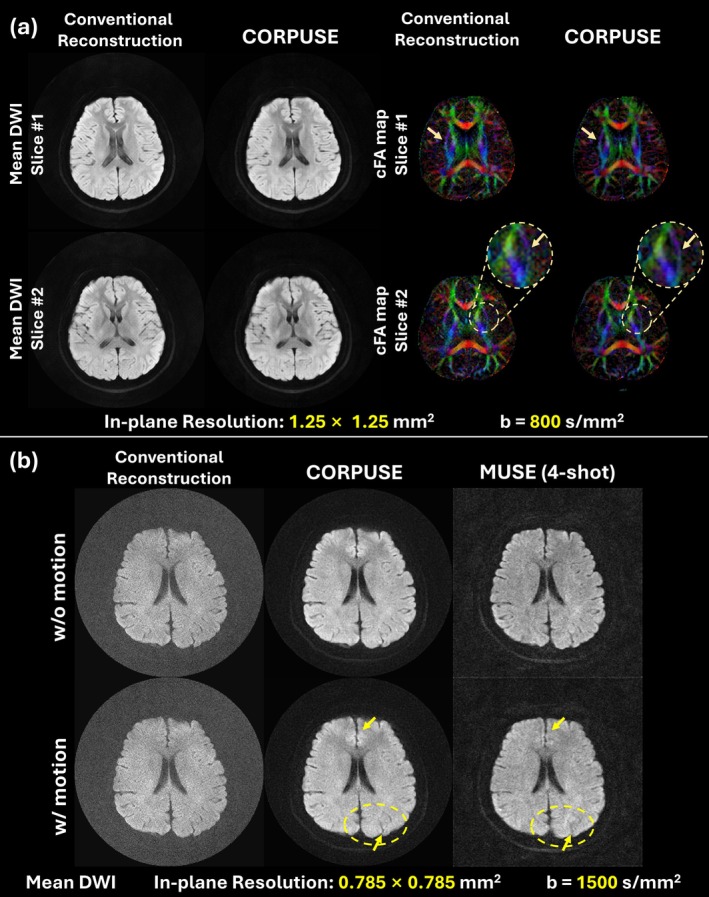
(a) DTI feasibility comparison using 8‐blade LAP‐EPI data acquired with 15 diffusion directions (*R* = 2). Mean DW images and cFA maps were computed from datasets reconstructed with either conventional PROPELLER‐EPI reconstruction or the CORPUSE framework. cFA maps derived from the CORPUSE‐reconstructed data exhibited superior noise suppression and improved delineation of white matter tracts, with noticeably sharper structural boundaries compared to those produced by conventional PROPELLER‐EPI reconstruction, particularly evident in the zoomed regions. (b) Comparison between conventional PROPELLER‐EPI reconstruction, CORPUSE framework, and four‐shot MUSE DWI under conditions with and without subtle head motion. CORPUSE yields sharper structural detail and reduced noise compared to conventional PROPELLER‐EPI reconstruction, while maintaining robustness to subtle motion and preserving fine anatomical features (yellow arrows). In contrast, four‐shot MUSE DWI exhibits pronounced motion‐induced blurring and diminished signal fidelity.
